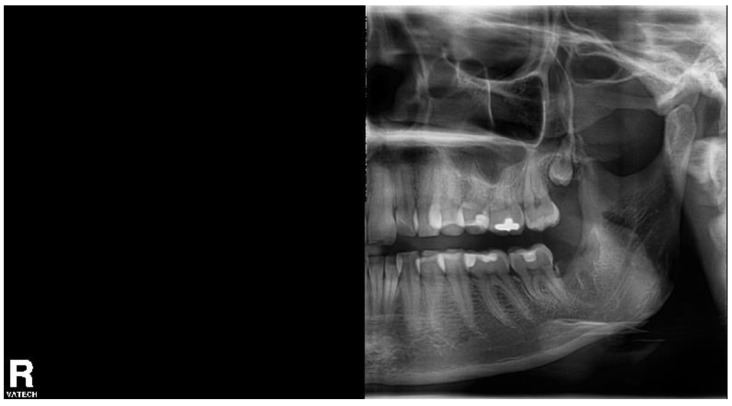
The half orthopantomogram of the left side of the patient 5. An empty socket of the tooth No. 38 is visible.
